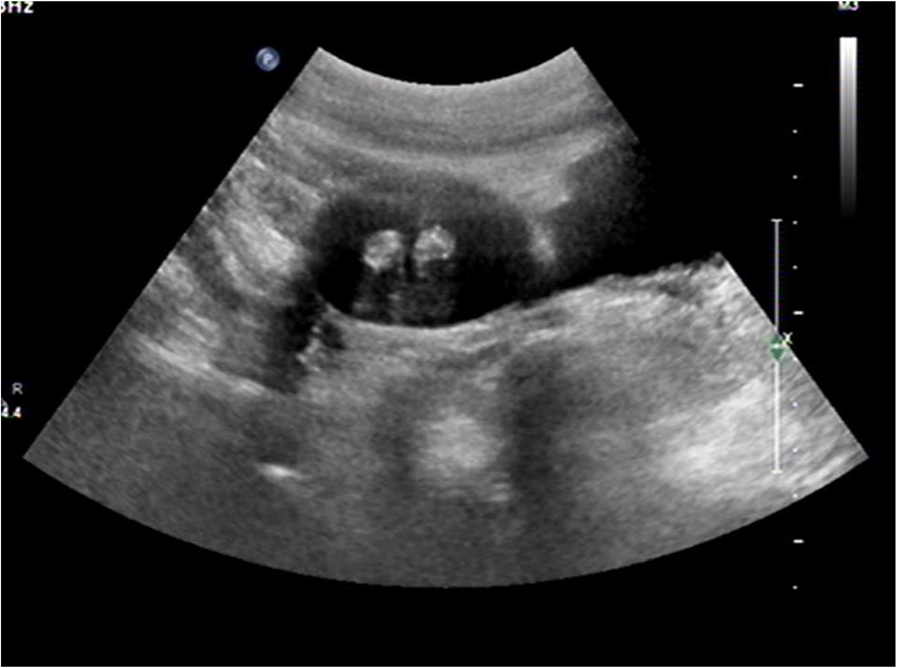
Two-dimensional ultrasound imaging showed a solid mass with clear boundary. It was hypoechoic with hyperechoic spots in the central part.
